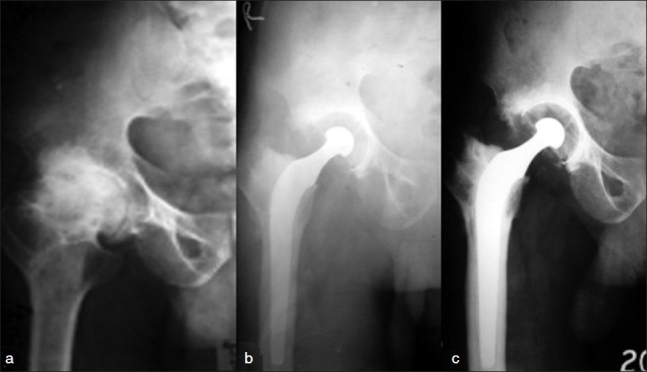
X-ray anteroposterior view of hip joint showing (a) AVN in a case of fracture neck femur treated with Mc Murrays osteotomy. (b) Cemented Charnley’s total hip arthroplasty was done. (c) Acetabular and femoral component cement loosening seen at 10 years followup.
